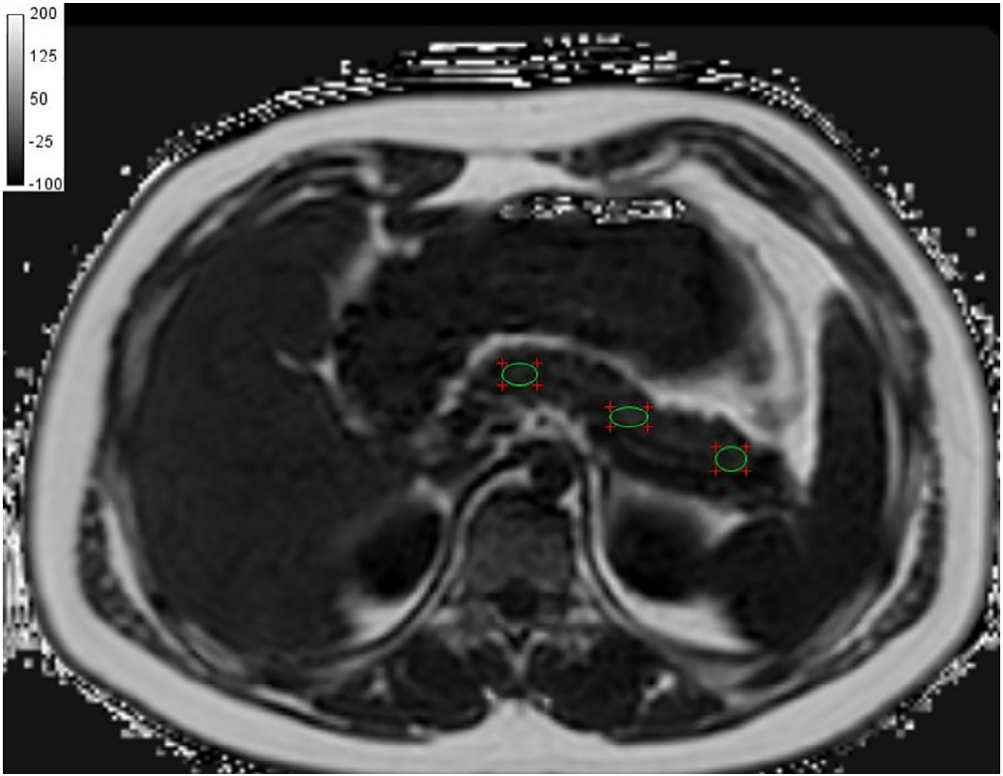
The regions of interest in the pancreas using chemical shift encoded MRI fat fraction image
